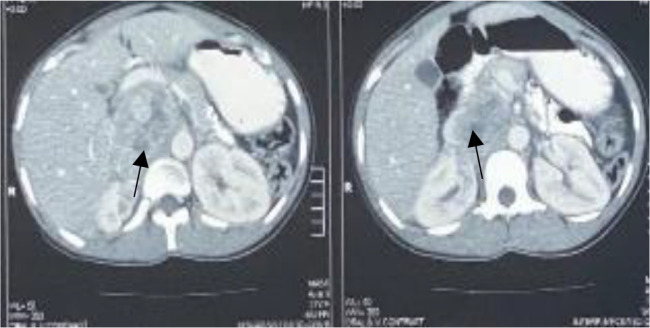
Abdominal CT scan; transverse section: showing a mass (7cm x 6cm) superior to the right kidney, with areas of necrosis (black arrows).
